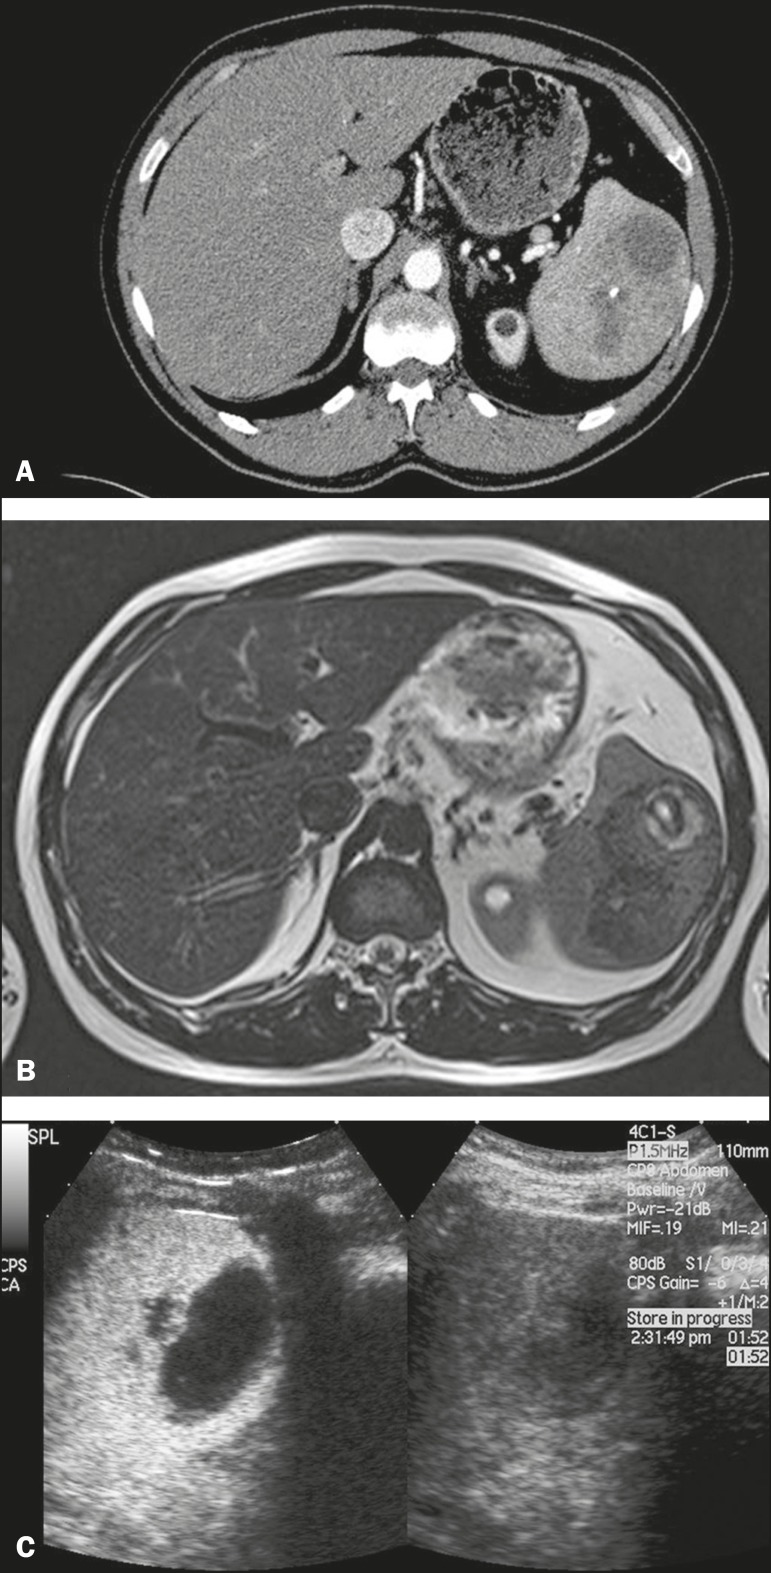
Splenic hamartoma. A: Venous-phase contrast-enhanced CT
